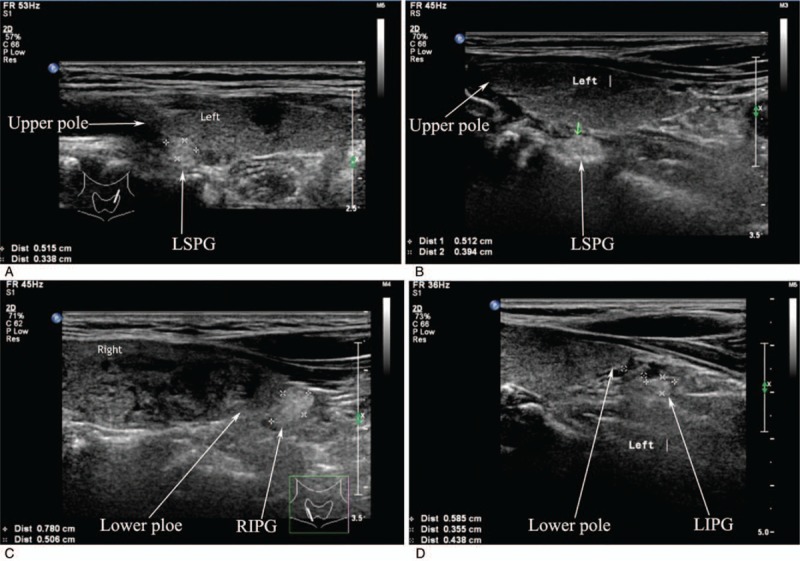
Longitudinal location of the normal parathyroid glands. (A) Ultrasonographic appearance of a LSPG located on the dorsal part of the upper third thyroid lobe; (B) ultrasonographic appearance of a LSPG located on the dorsal part of the middle third thyroid lobe; (C) Ultrasonographic appearance of a RIPG located on the dorsal part of the lower third thyroid lobe; (D) Ultrasonographic appearance of a LIPG placed 0.438 cm below the lower thyroid lobe. Longitudinal scan: LIPG = left inferior parathyroid gland; LSPG = left superior parathyroid gland; RIPG = right inferior parathyroid gland.
